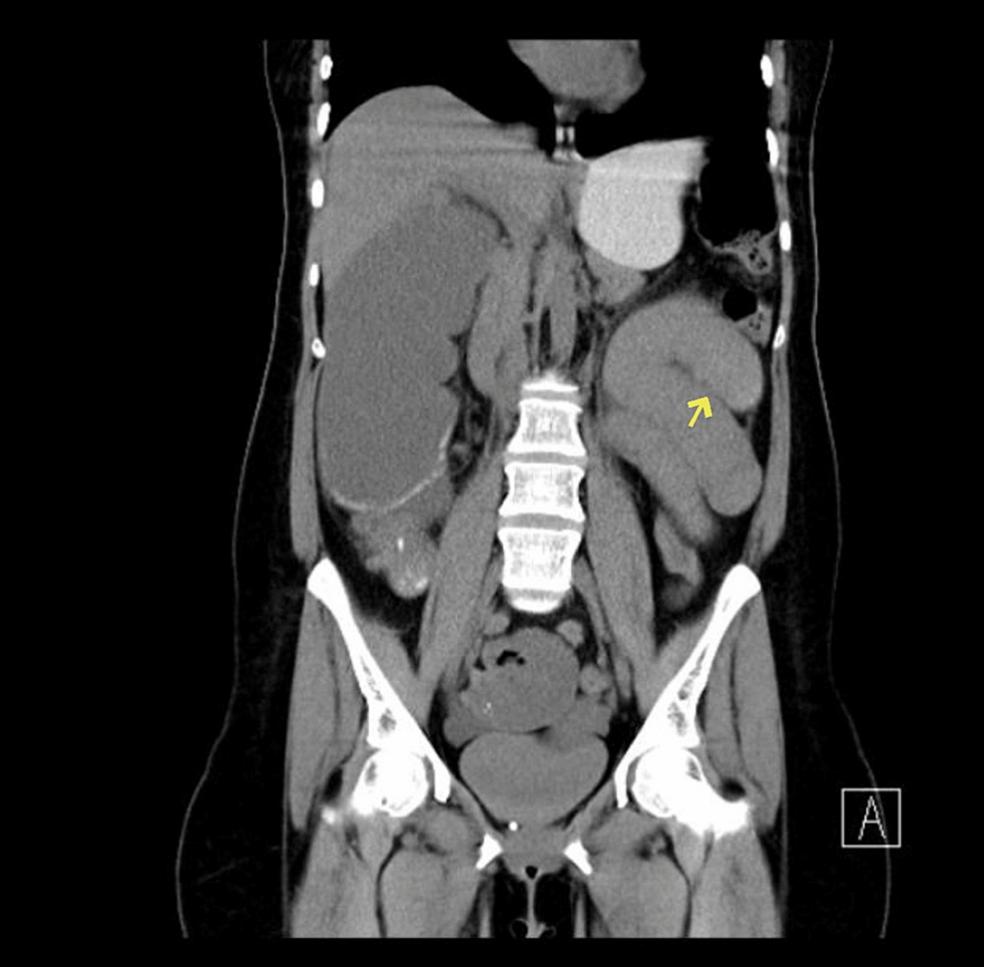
Case 1 - pre- and post-enhanced CT abdomen with oral and rectal contrast. It demonstrated a complete small and large bowel obstruction. At the level of the splenic flexure, there was small annular constricting thickening with transition zone measuring 3.2 cm.
